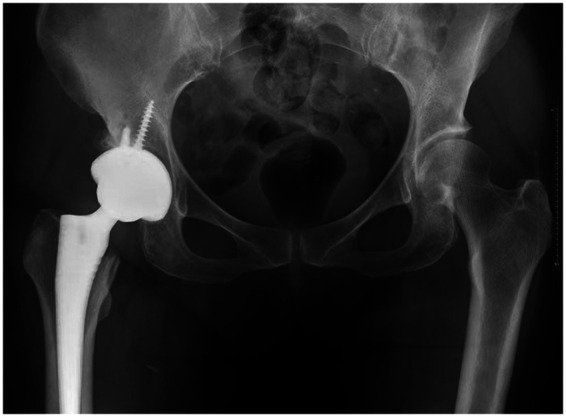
Postoperative x-ray indicated that the joint prosthesis was installed properly.
